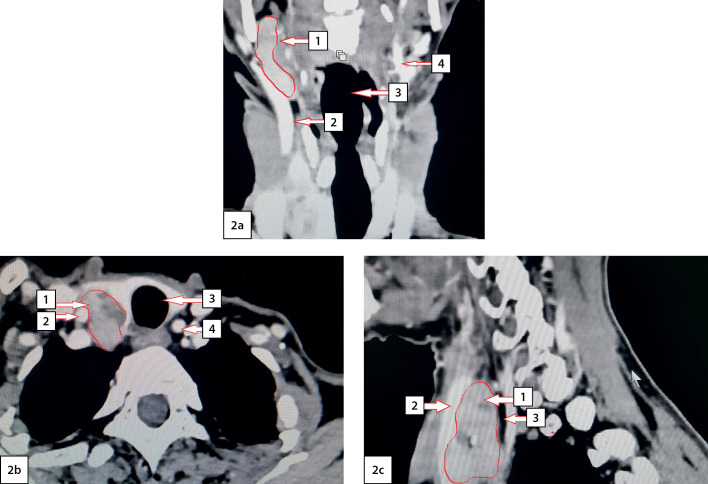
Figure 2. Computed tomography of the neck with angiography: 2a — frontal view; 2b — axial view; 2c — sagittal view. Arrows indicate anatomical structures: 1 — the upper right parathyroid gland; 2 — right common carotid artery; 3 — trachea; 4 — left common carotid artery.
